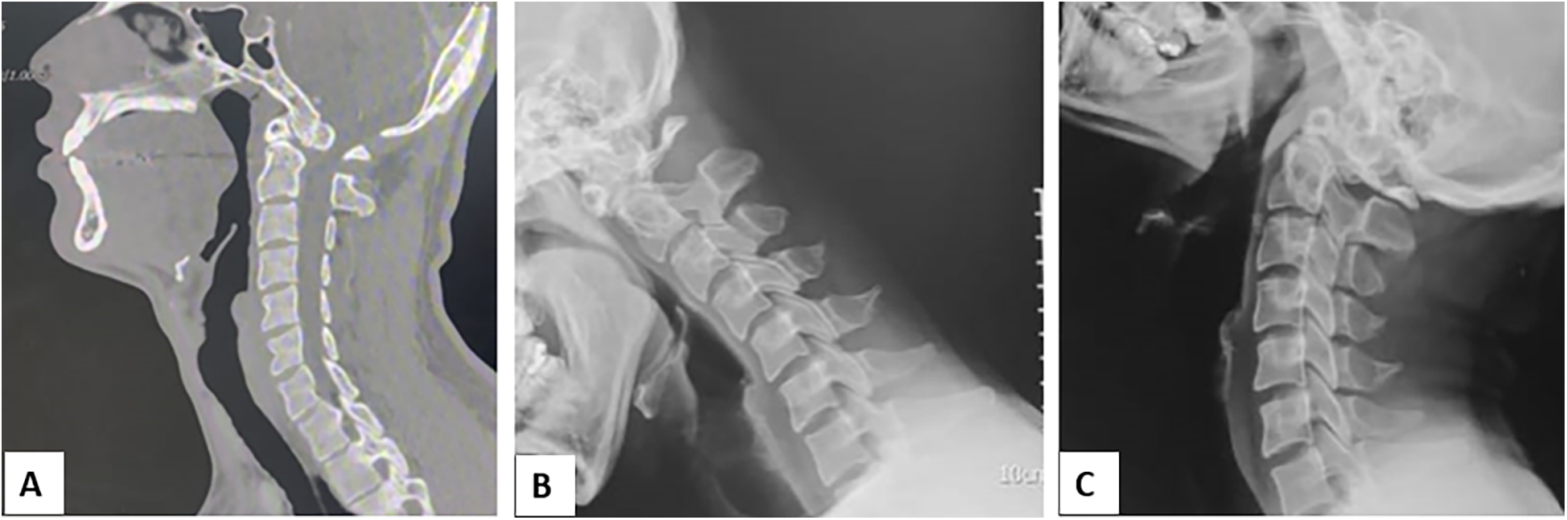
(A) Mid-sagittal computed tomography of cervical spine showing unfused C2 body with the odontoid process (B,C) and dynamic radiographs of Cervical spine showing C1–C2 instability. Three side-by-side medical images of the cervical spine. Image A is a CT scan showing detailed bone structure. Image B is an X-ray with improved contrast, highlighting vertebrae. Image C is a darker X-ray showing alignment.
